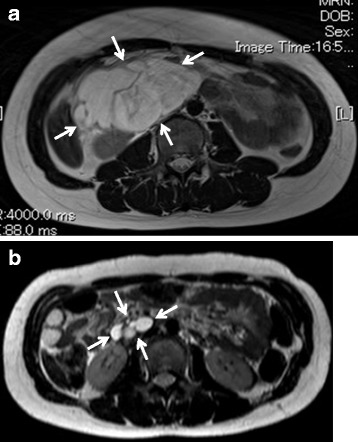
At the first admission, magnetic resonance imaging (MRI) showed that a large multicystic mass (*arrow*) revealed hyperintense on T2 images and did not contain any fat tissue (a). Six months after TJ-28 medication, MRI showed that the tumor size decreased markedly to 3.5 × 1.5 × 1.2 cm in size (*arrow*, b)
